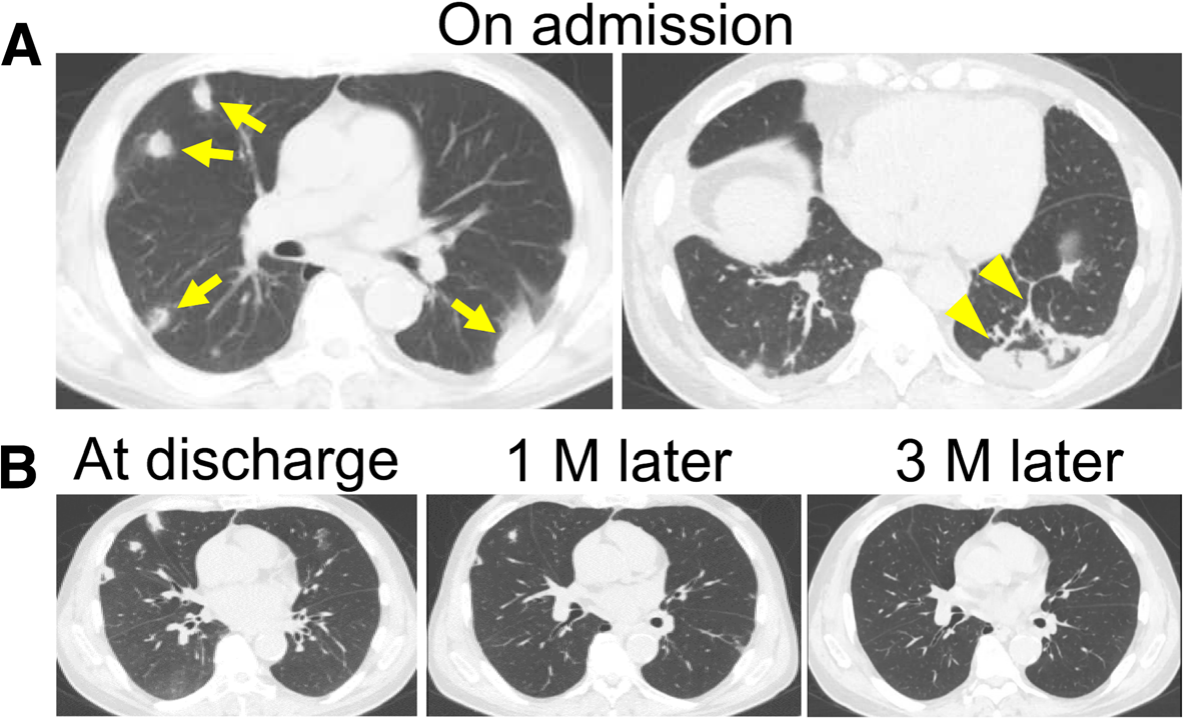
CT images of septic pulmonary emboli. a Chest CT images on admission. Arrows indicate septic embolic lesions. Arrowheads indicate a feeding vessel sign and a wedge-shaped peripheral lesion. b Follow-up images at discharge, 1 month and 3 months after the discharge
